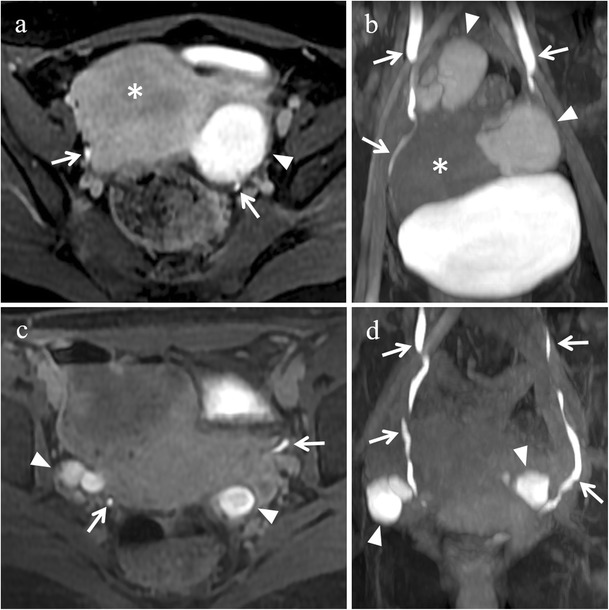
Ureter visualization with intravenous gadolinium and variability of the ureteral course in two different patients with bilateral endometriomas. 27-year-old woman (a, b). (a) Axial contrast-enhanced fat-suppressed T1-weighted image (acquired about 10 min after contrast administration) and (b) corresponding coronal MIP image. The right ureter (white arrows) courses along the lateral margin of the right endometrial cyst (white arrowhead) and the uterus (white *), the left ureter (white arrows) courses along the posterior margin of the left endometrioma (white arrowheads). 35-year-old woman (b, c). (c) Axial contrast-enhanced fat-suppressed T1-weighted image (acquired about 10 min after contrast administration) and (d) corresponding coronal MIP image. The right ureter (white arrows) courses medially to the omolateral endometrial cyst (white arrowheads), the left ureter (white arrows) courses laterally to the omolateral endometrioma (white arrowheads). Preoperative knowledge of ureteral course is important in order to prevent iatrogenic injuries to the ureters
